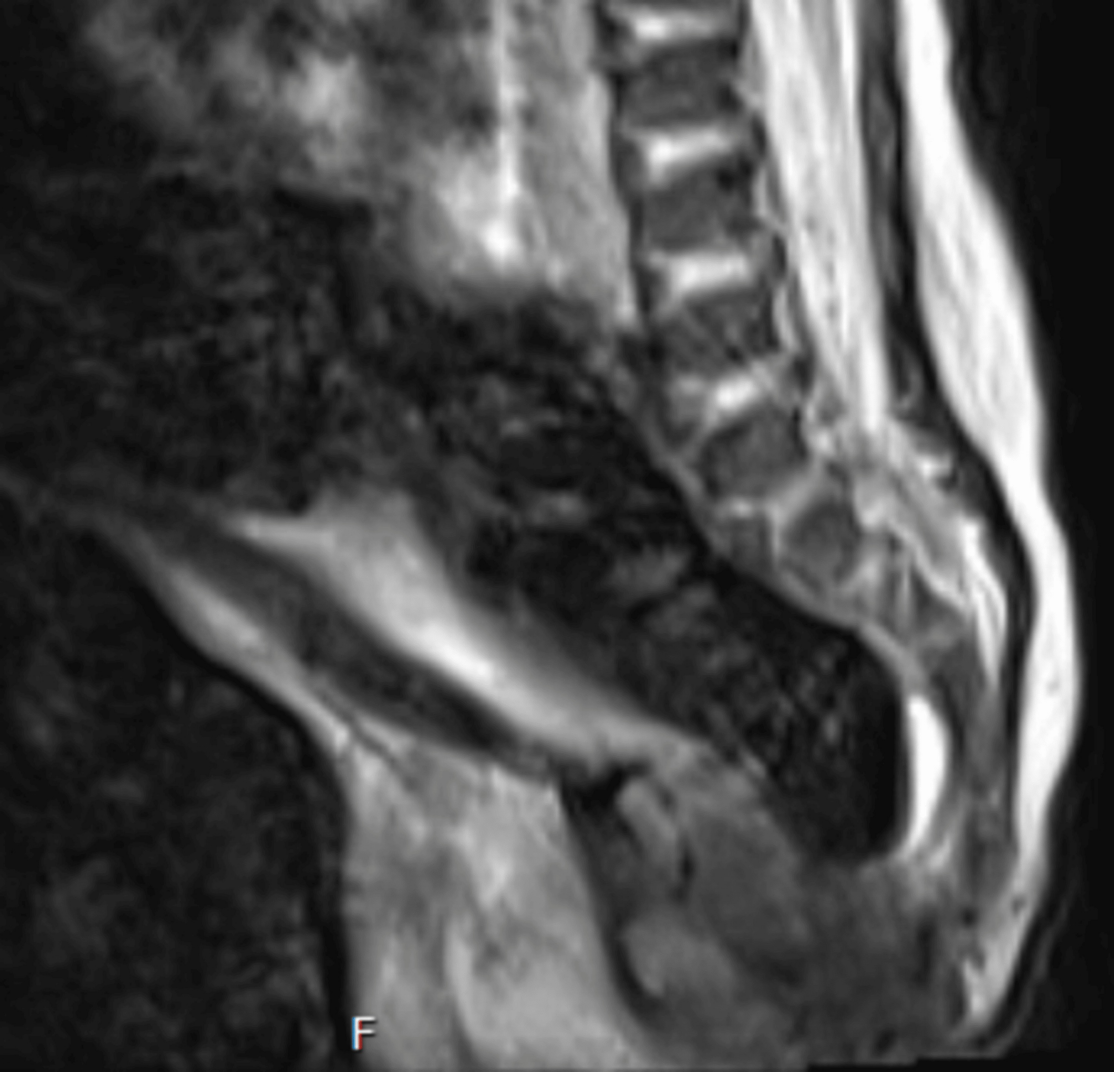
MRI lumbar spine (T2 sagittal) demonstrating sacral agenesis, low-lying conus medullaris, and anal atresia/imperforate anus (motion artifact present).
